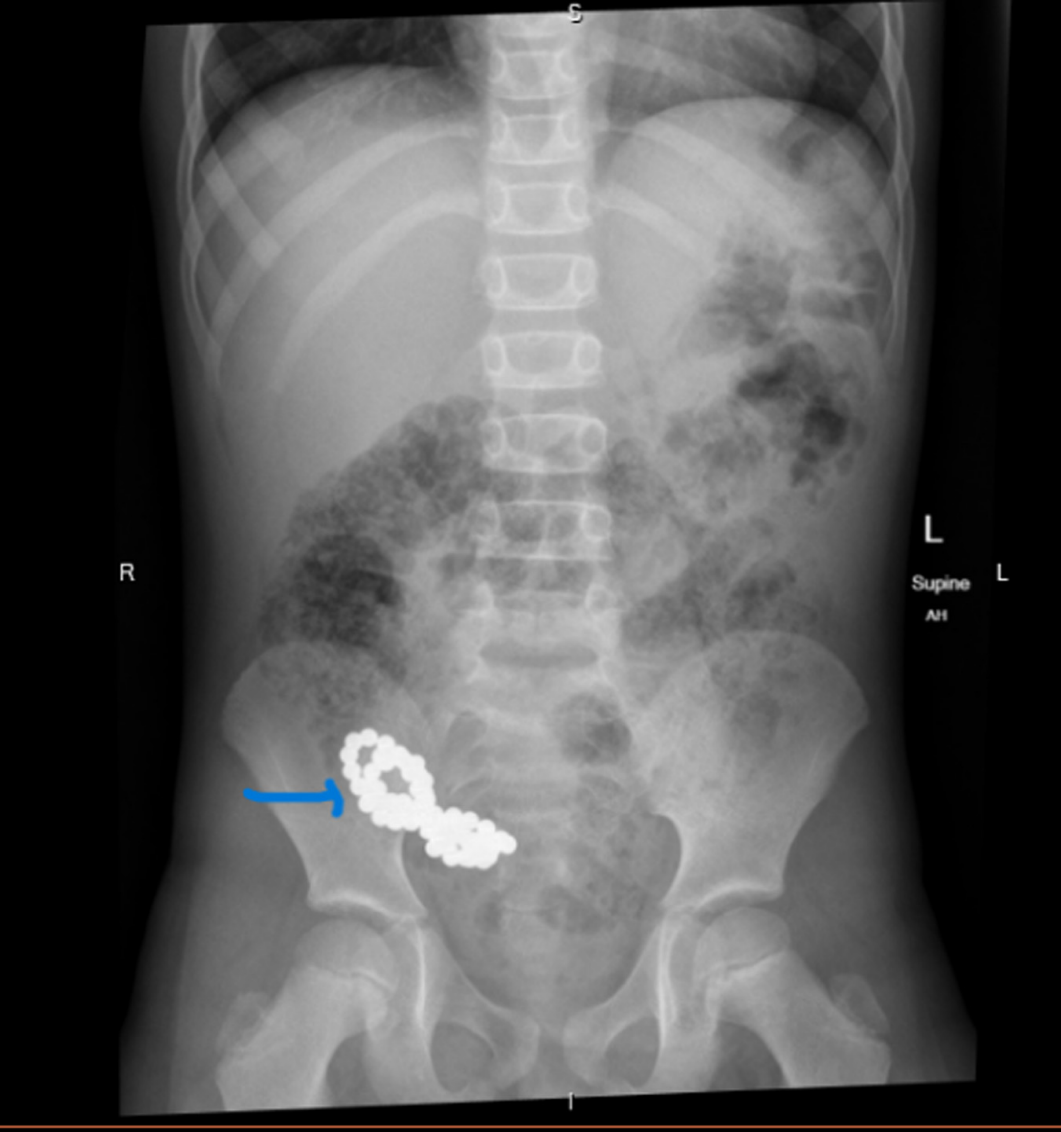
Supine radiograph of the abdomen showing multiple rare-earth magnets (blue arrow).
